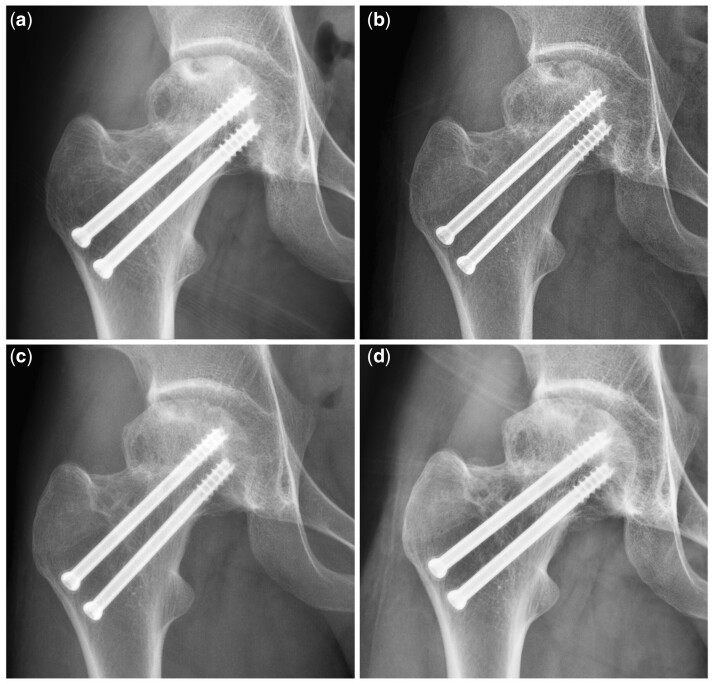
Children with TFHN at collapsed stage showed a satisfactory outcome in a long-term follow-up when their hips were in a stable condition (without subluxation). A male patient of 14 years old, with right FNF, treated with Kirschner wires. TFHN (JIC classification C2) was diagnosed 1 year after FNF (a). During observational treatment, radiographs showed progression of femoral head collapse after 1 year follow up, but still without subluxation (b). At 2 years follow-up, it still showed no significant progression of collapse and deformity (c). After 5 years, the patient still got good outcome according to Ratliff’s clinical criteria, with mild hip deformity (Stulberg classification II) and mild hip degeneration (Tönnis grade I).
